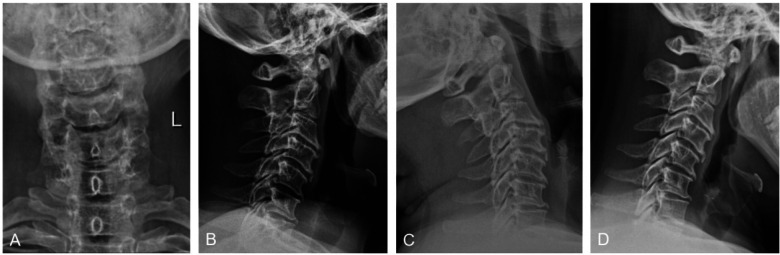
Preoperative cervical x-ray. (A) The anteroposterior view of the cervical vertebra demonstrates signs of degeneration, with a loss of normal curvature. (B) The lateral view reveals further cervical vertebral degeneration and a straightened physiological curvature. (C,D) Dynamic imaging (flexion and extension views) shows no signs of cervical vertebral instability, although degenerative changes are evident in these positions.
